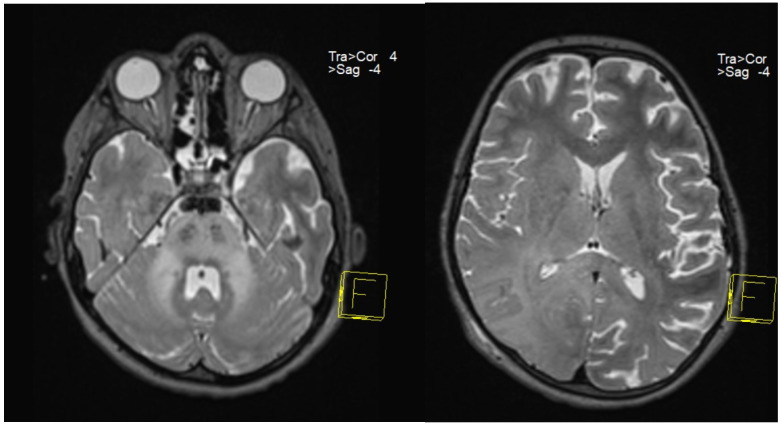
MRI performed on case 6: Symmetrical generalized T2 hyperintensity at the pontine level and middle cerebellar peduncles, with additional involvement in the supratentorial temporo-parieto-occipital regions and right frontal cortico-subcortical areas, in the corpus callosum and left parieto-occipital region.
